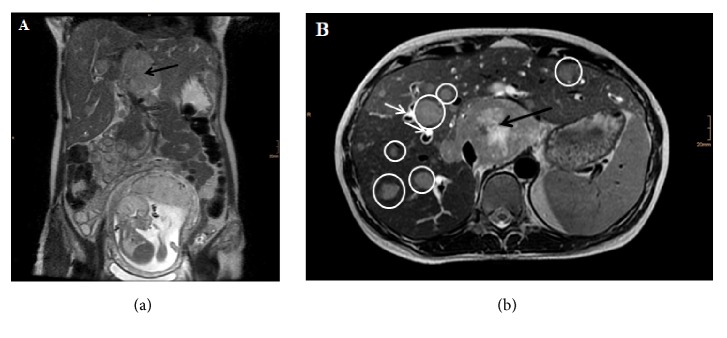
Magnetic resonance imaging (MRI) shows an enlarged liver with central tumor (black arrow), dilated biliary ducts (white arrows), and disseminated metastases (circles) of a pregnant woman at 20 weeks 4 days of gestation. (a) coronal, (b) axial view.
